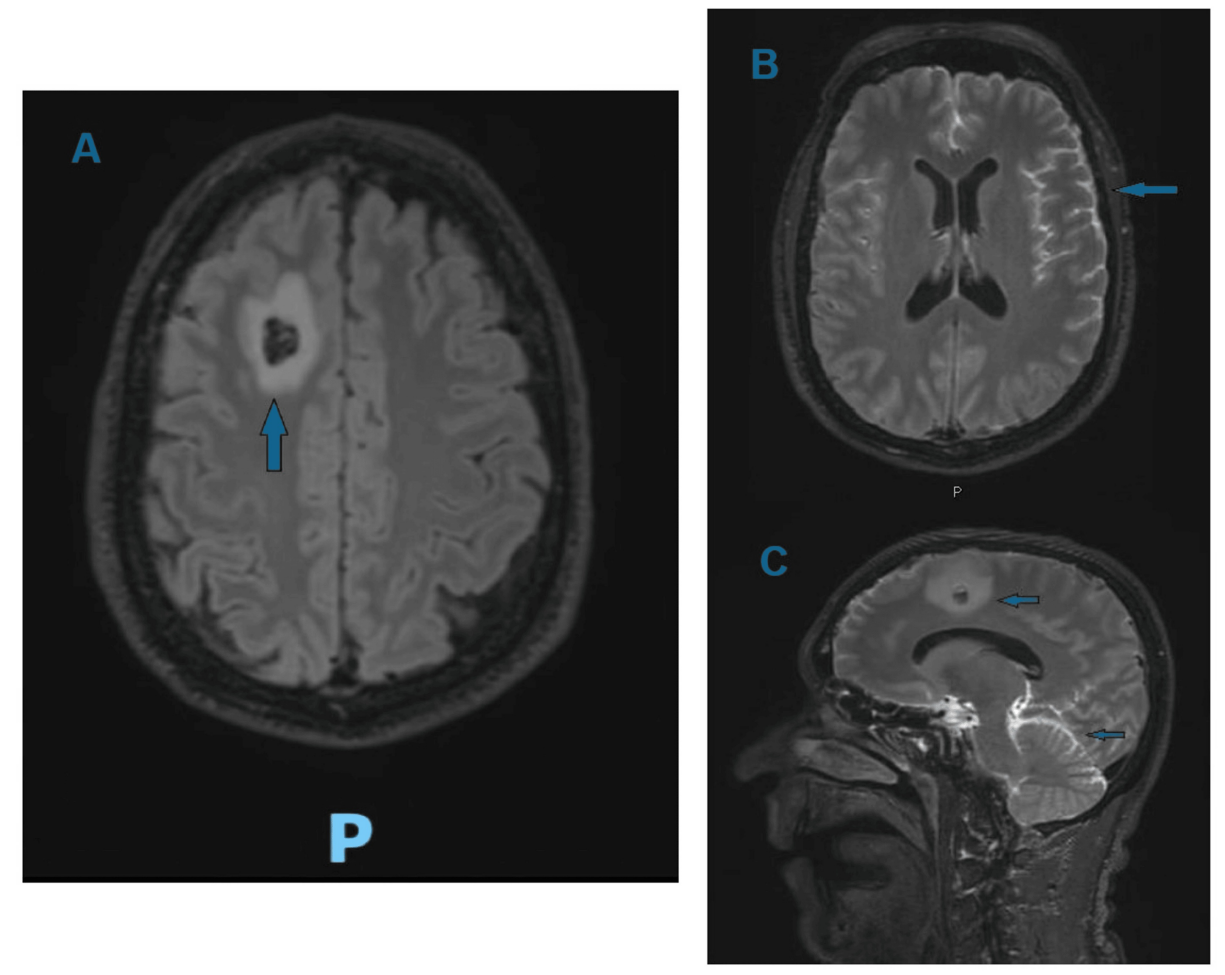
T2-weighted sequence MRI showing an abscess in the right frontal region (A and C) and leptomeningeal enhancement (B and C).
